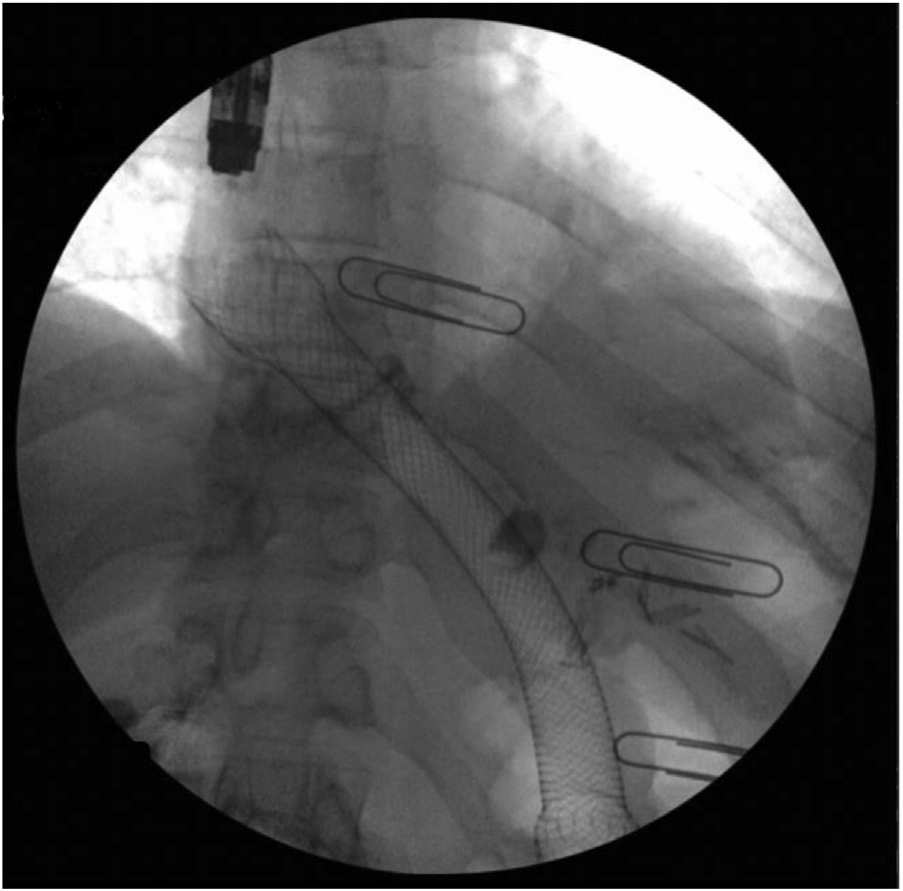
Radiograph showing the initial esophageal self-expanding metal stent remains in adequate position.
